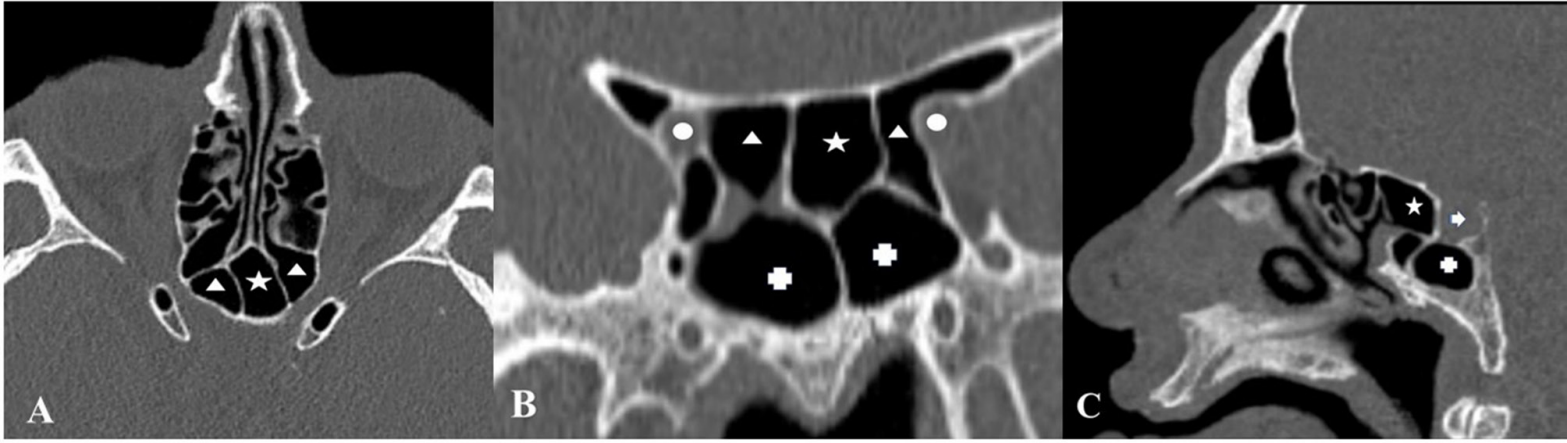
SSC is demonstrated in all three planes. Onodi cells (triangle) attach to the optic nerve (circle) on both sides (Axial (A) and Coronal (B) CT scans). A sphenoid sinus (cross) lying inferior to an SSC (Sagittal (C) and Coronal scans) is shown. A Coronal-sagittal projection of the SSC (Arrow: pituitary gland).
